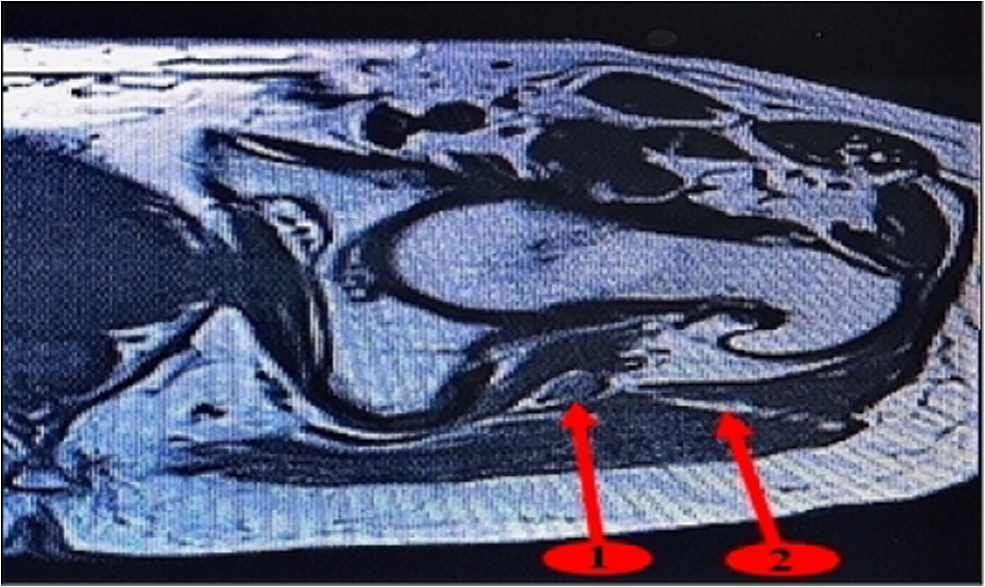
Muscular interstice between the posterior and anterior heads of the gluteus maximus (spot 2) and its proximity to the sciatic nerve (spot 1) (MRI image)
